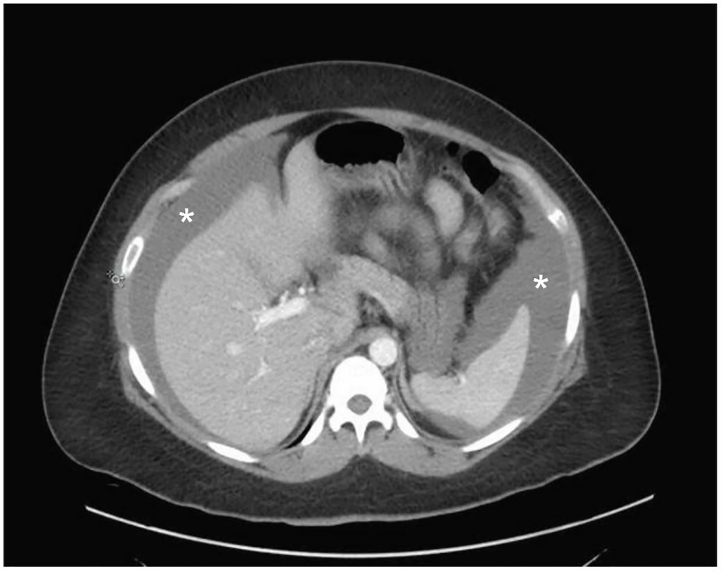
Abdominal computed tomography, cross section, demonstrating location of hemoperitoneum (asterisks).
